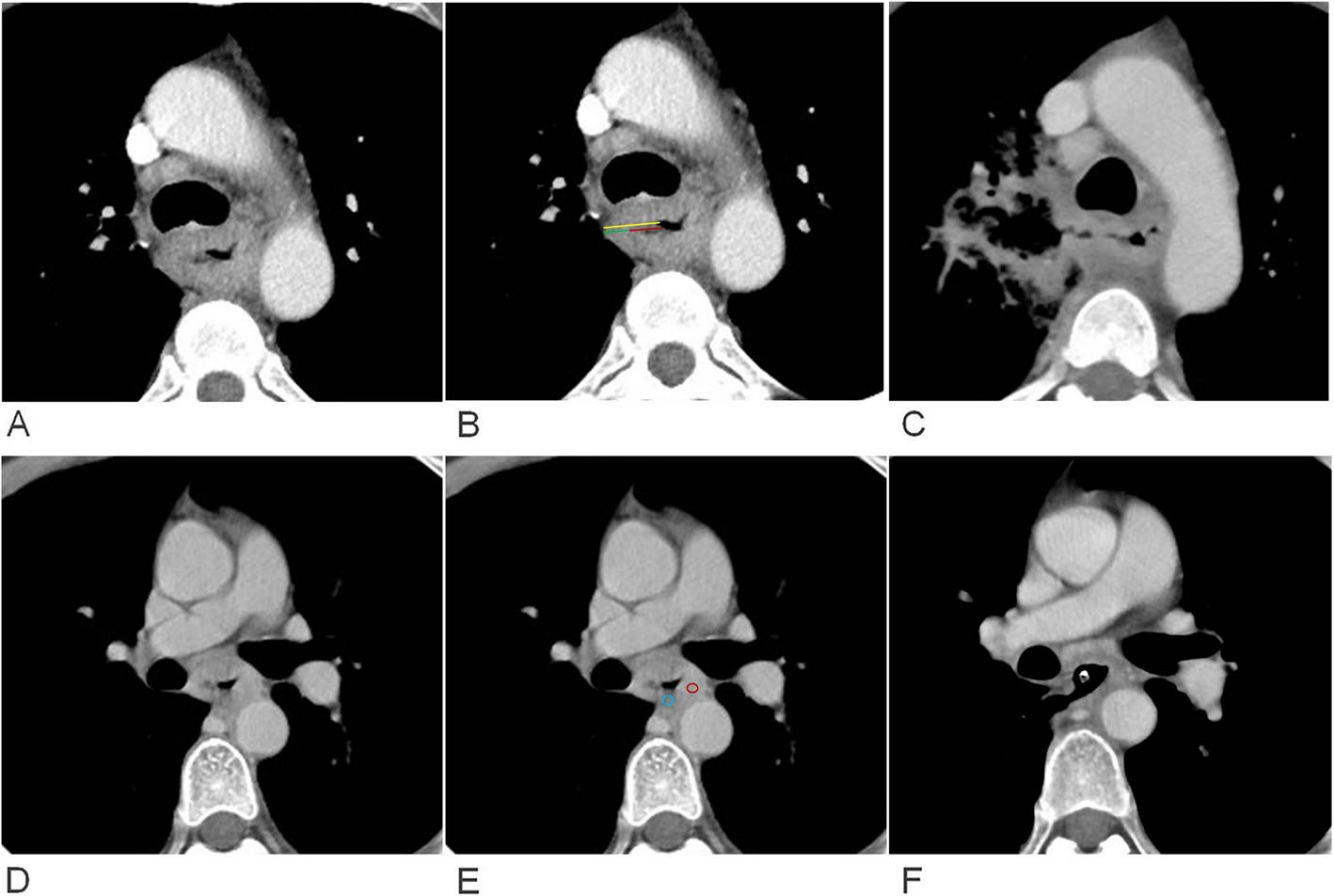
Axial enhanced CT at baseline examination showing ROI delineation and deep ulcer measurement. (A-C) CT images in a 42-year-old woman with esophageal cancer. A CT image showed an esophageal mass with a deep ulcer in the right wall. B The same CT image showed the measurement of the depth of deep ulcer (red line) at 10 mm, residual esophageal wall (green line) at 7 mm and the thickness of adjacent wall to ulcer (yellow line) at 17 mm in the ulcer layer. The ratio of ulcer depth to adjacent tumor thickness of 58.8% and tumor thickness of 17 mm could predict fistula formation after treatment. C The esophageal fistula occurred in the region of the deep ulcer after three chemotherapy cycles and radiotherapy. D-F CT images in a 50-year-old man with esophageal cancer. D CT image showed an esophageal mass with a thickness of 15 mm. E The highest (red circle; 119 HU) and lowest enhancement (blue circle; 20 HU) zones, the ratio of minimum to maximum enhanced CT value of 16.8% and a tumor thickness of 15 mm could predict fistula formation after treatment. F Esophageal fistula occurred in the region of the lowest zone after three chemotherapy cycles and radiotherapy
